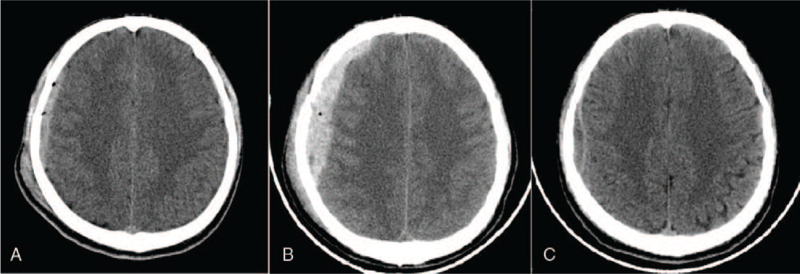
(A) First CT scan showing acute epidural hematoma in the right forehead. (B) Second CT scan, obtained at admission, showing that the hematoma had enlarged. (C) Follow-up CT scan obtained 23 days after embolization showing the hematoma had significantly reduced. CT = computerized tomography.
